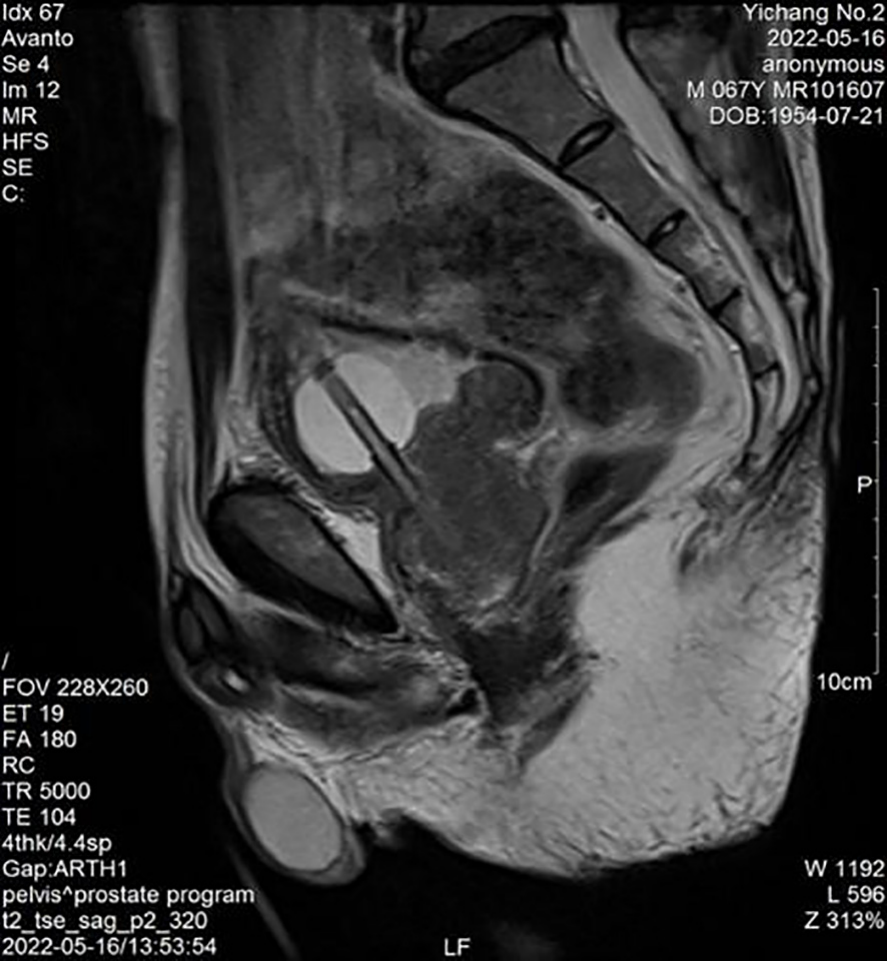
Prostate peripheral zone abnormal signals (MRI).
